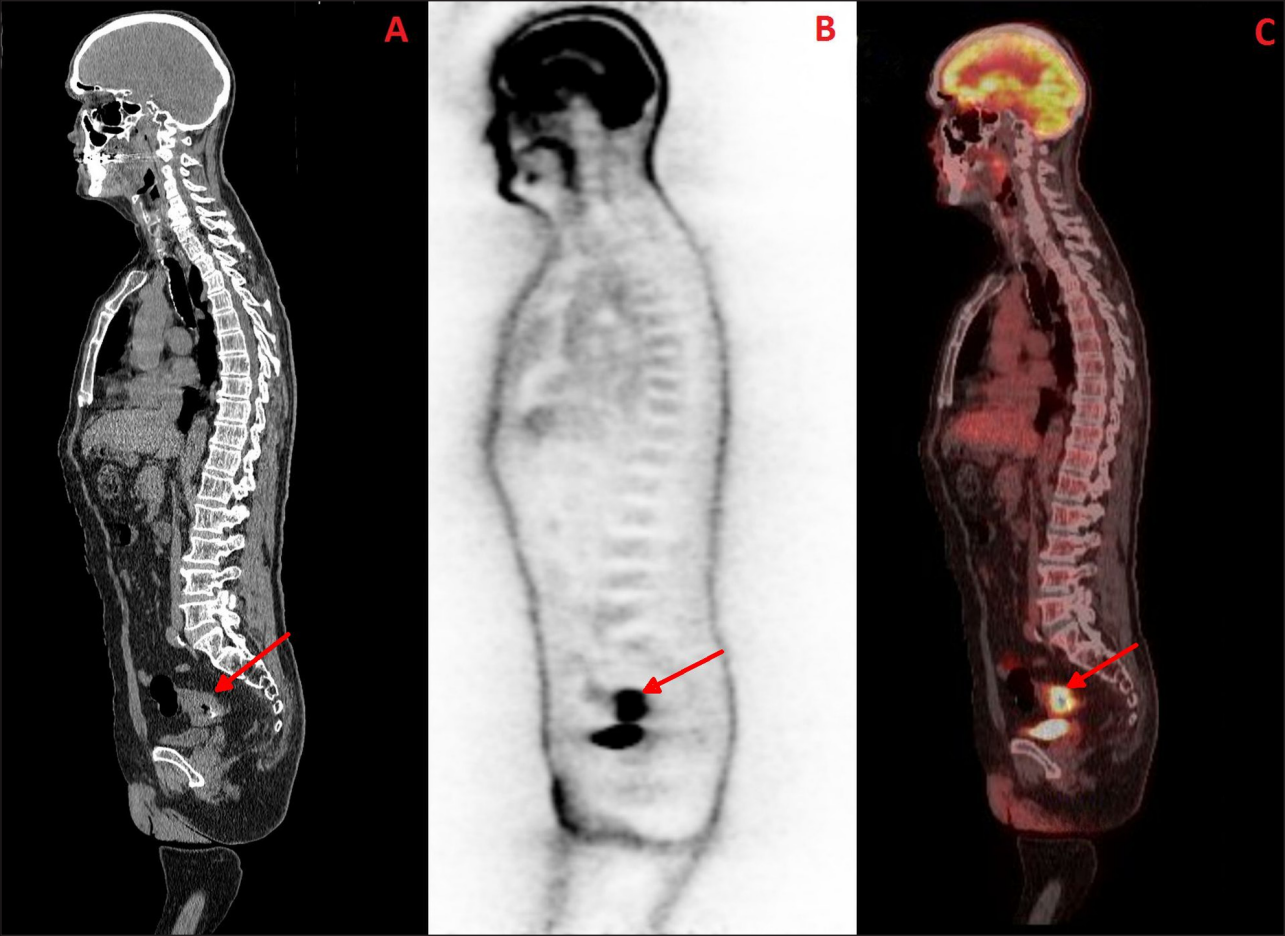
Sagittal plane; A- CT image; B- PET image; C- Fused PET-CT image.
